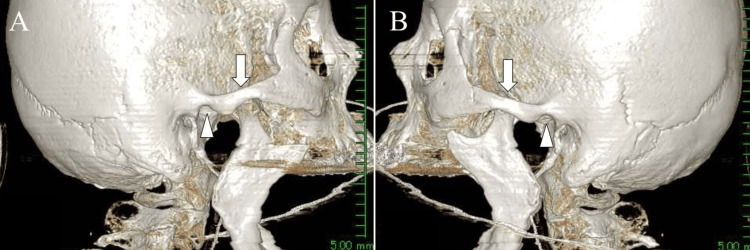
Three-dimensional CT eight weeks after onset: three-dimensional CT demonstrating empty glenoid fossae in both temporomandibular joints (arrowheads) with anteriorly displaced condyles (arrows). (A: right side, B: left side).
